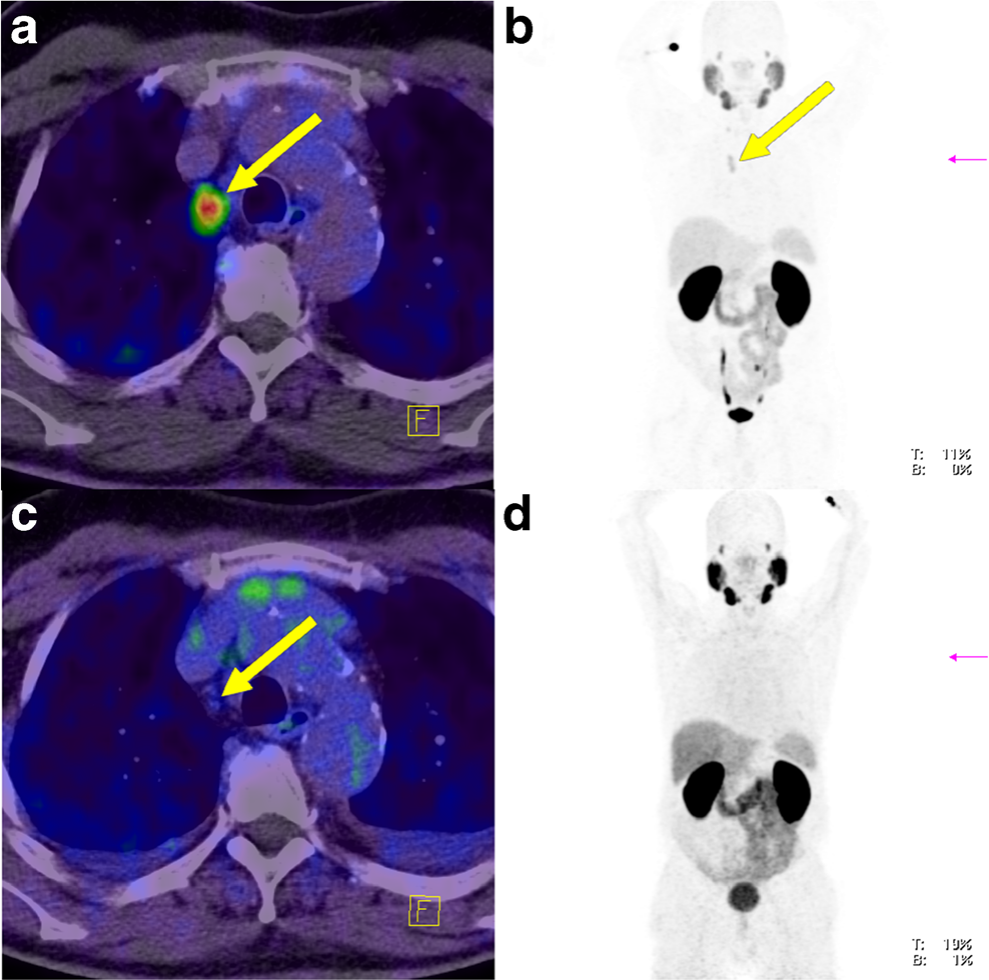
Lymph node metastasis in an example patient (patient 8; *yellow arrows*) without ADT (a, b) and during ongoing ADT with a complete PSA response (c, d) 190 days after the first scan. During ongoing ADT, both, tracer uptake intensity and tumour volume significantly decreased. a Fused PET and CT image without ADT. b Maximum intensity projection image of the PET data without ADT. c Fused PET and CT image during ongoing ADT. d Maximum intensity projection image of the PET data during ongoing ADT
